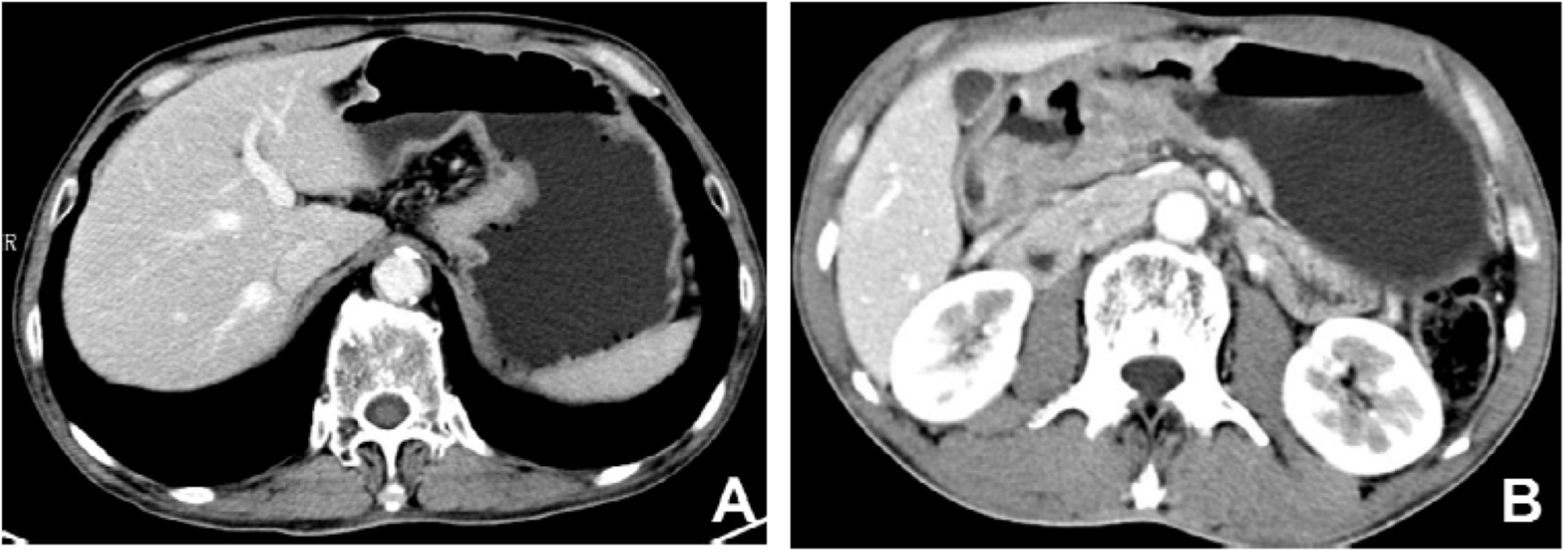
Contrast-enhanced computed tomography images of non-mucinous gastric carcinoma (A) A 67-year-old male with cardiac carcinoma showed obviously homogenous enhancement without calcification. (B) A 56-year-old male with lesion in gastric antrum showed obviously heterogeneous enhancement without calcification.
